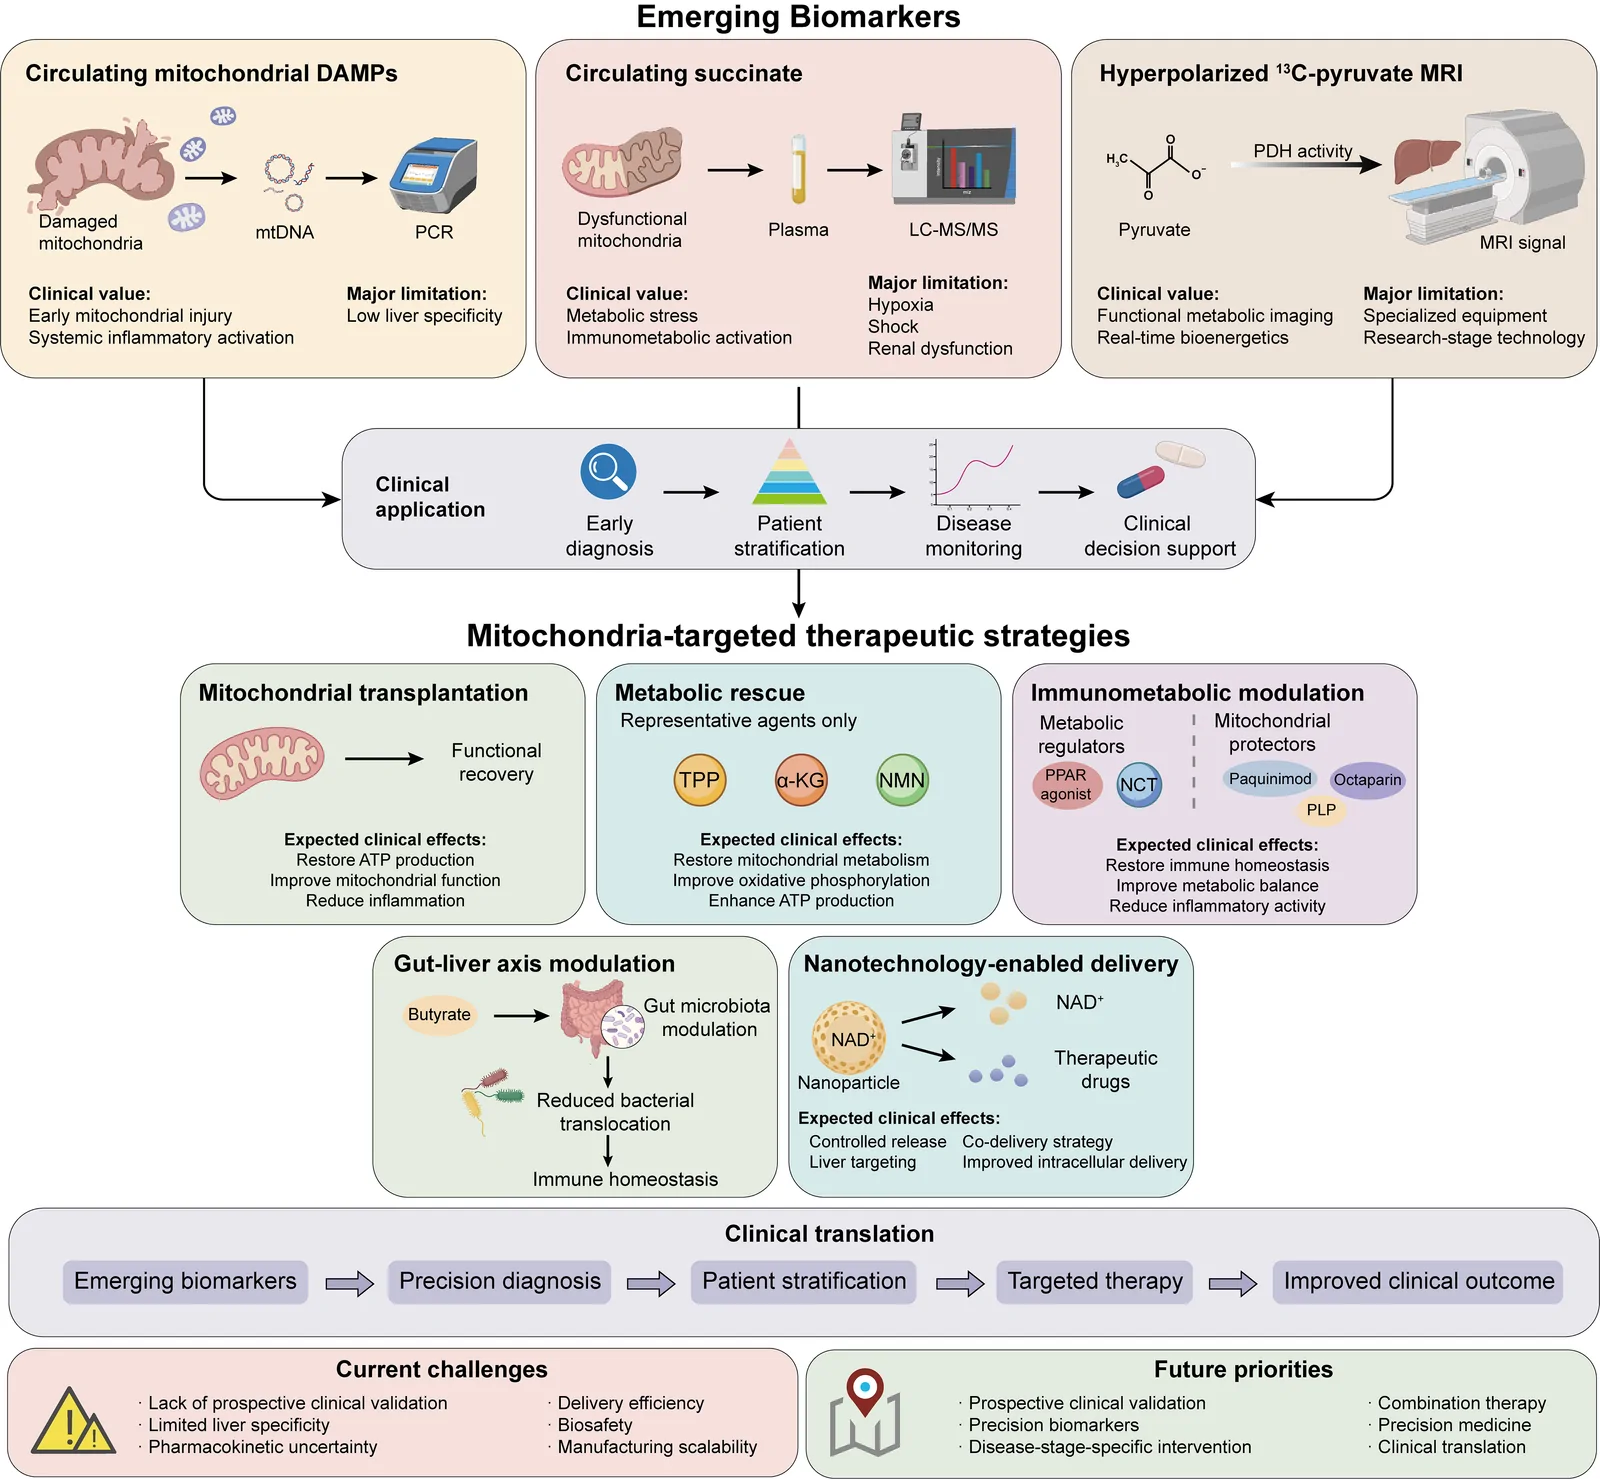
Emerging mitochondrial biomarkers and mitochondria-targeted therapeutic strategies for clinical translation. Emerging biomarkers of mitochondrial dysfunction include circulating mitochondrial DAMPs (e.g., mtDNA), metabolic intermediates (e.g., succinate), and functional metabolic imaging using hyperpolarized ^13C-pyruvate MRI. These biomarkers reflect mitochondrial injury and metabolic reprogramming and enable early diagnosis, patient stratification, disease monitoring, and clinical decision support. Mitochondria-targeted therapeutic strategies include mitochondrial transplantation, metabolic rescue, immunometabolic modulation, gut–liver axis regulation, and nanotechnology-enabled delivery systems. Collectively, these approaches link mitochondrial dysfunction to metabolic and immune dysregulation, providing a translational framework from biomarker identification to targeted intervention and improved clinical outcomes in sepsis-induced liver injury.
